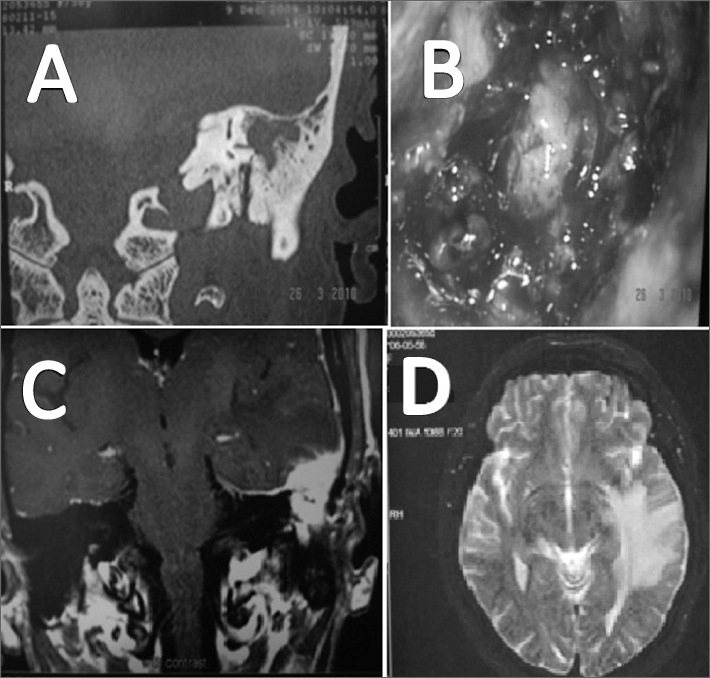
A: Temporal bone CT scan showing a lesion eroding the lateral semicircular canal; B: Tumor aspect during surgery; C: Contrasted skull T1 MRI showing left temporal lobe tumor invasion; D: T2 skull MRI in which we see an important cerebral edema.
